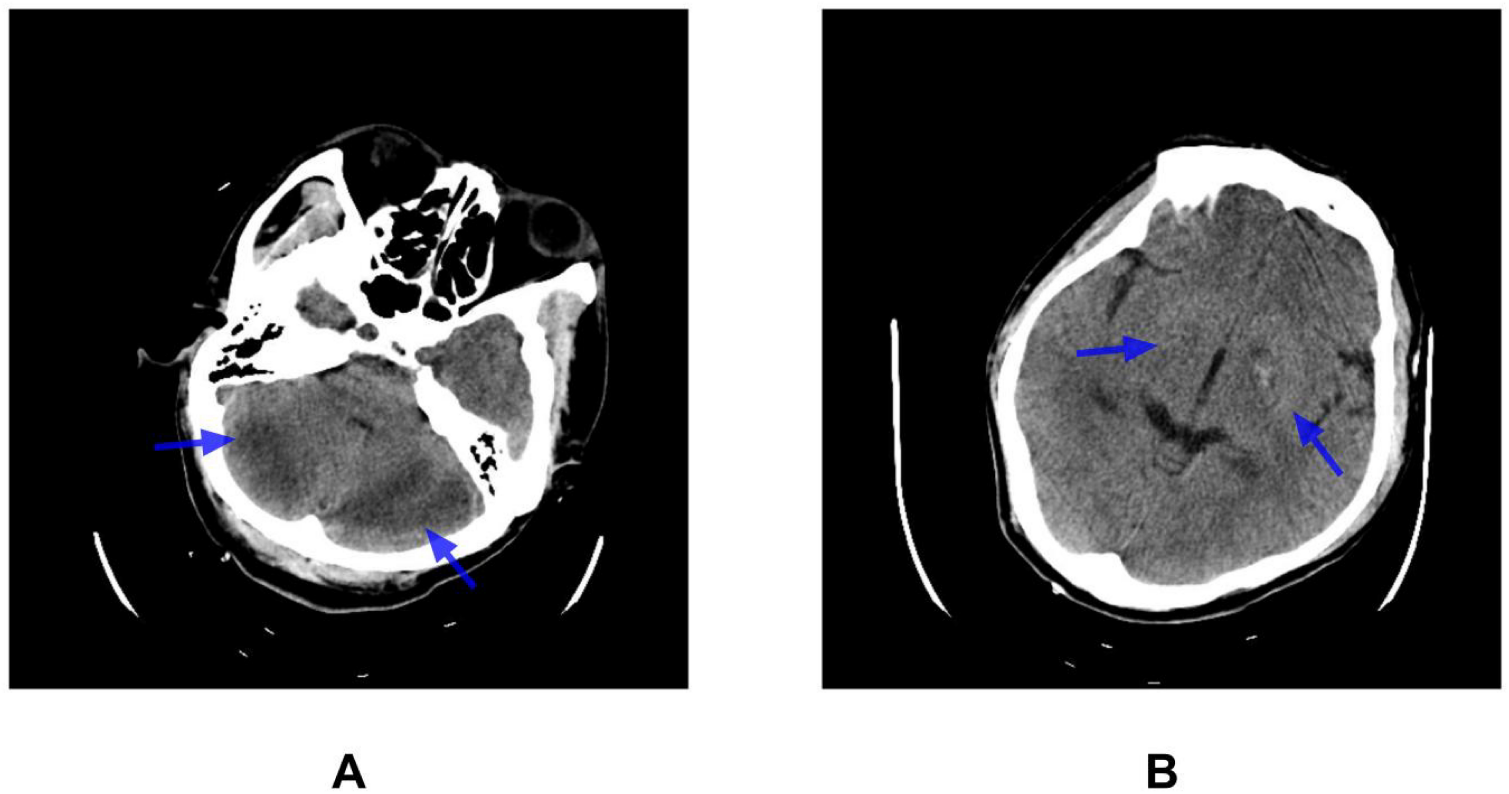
Brain CT findings (day 3). CT scan showing (A) bilateral hypodense lesions in the cerebellar hemispheres and (B) mixed-density lesions in the bilateral basal ganglia, as indicated by the blue arrows.
